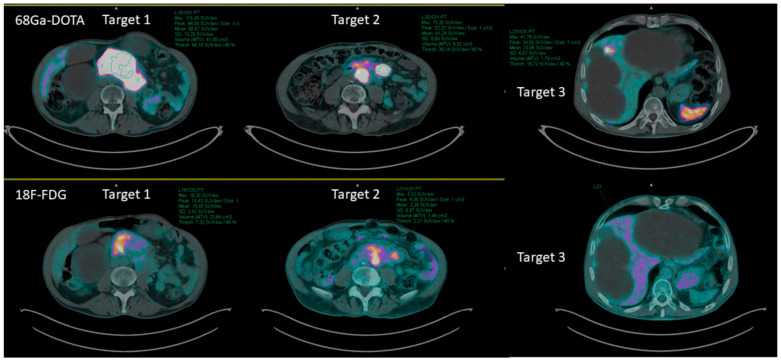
The figure shows the selection process for the target lesions on baseline FDG and [^68^Ga]Ga-DOTATOC PET/CT in a patient of the series. Target 1 was selected on the pancreatic primary lesion, and target 2, on a loco-regional lymph node metastasis. In this case, target 3 was identified only on [^68^Ga]Ga-DOTATOC PET/CT, as the liver metastasis showed no FDG avidity. However, the same target was re-evaluated on follow-up FDG PET/CT to identify any possible glycometabolic variation.
